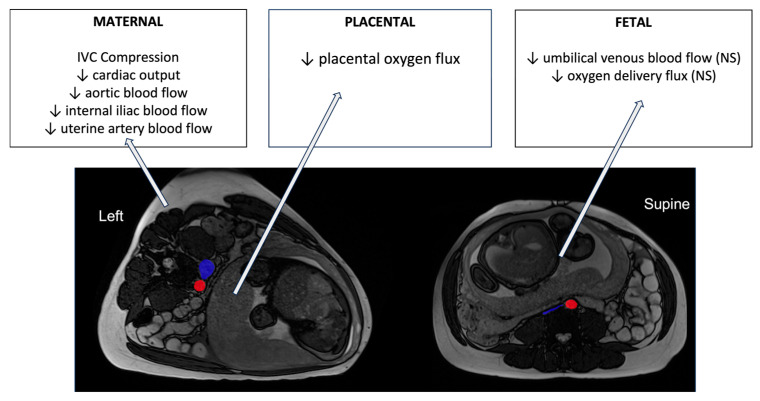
A summary of the changes in blood flow and oxygen transfer parameters in healthy pregnancies in late gestation derived from MRI imaging studies reviewed here. MRI images are shown in axial planes, using radiological convention for axes. The IVC and aorta are highlighted in blue and red, respectively. NS = non-significant changes.
